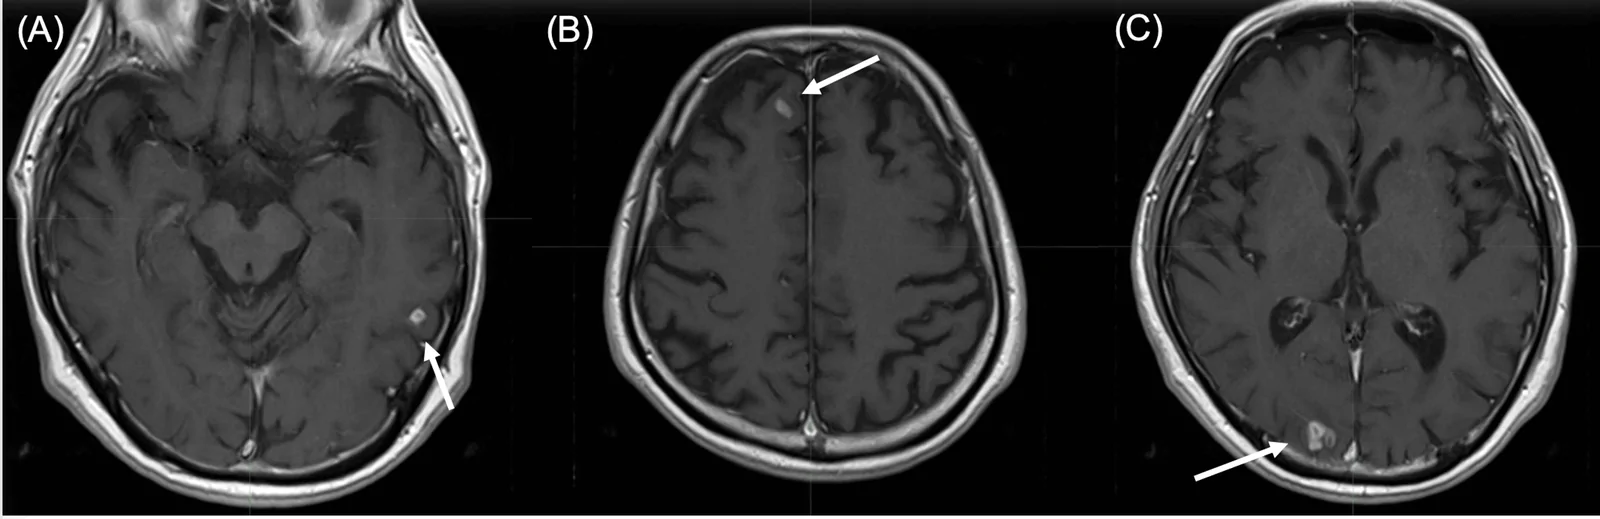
Contrast-Enhanced Brain MRI Showing Multiple Cerebral Abscesses Contrast-enhanced axial T1-weighted brain MRI obtained 27 days after starting radiotherapy. (A-C) Multiple variably sized ring-enhancing nodules are present in both cerebral hemispheres, consistent with cerebral abscesses (arrows).
